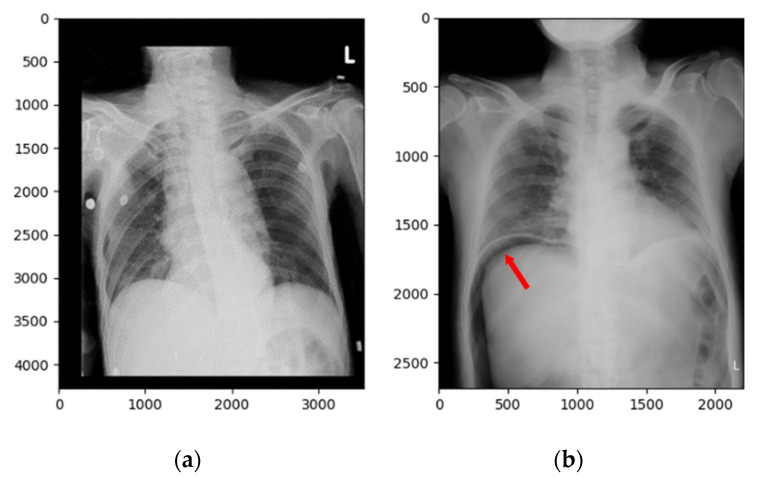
Frontal chest X-ray images of (a) a negative (normal) case and (b) a positive (pneumoperitoneum) case. The red arrow shows subphrenic free air, indicating pneumoperitoneum.
